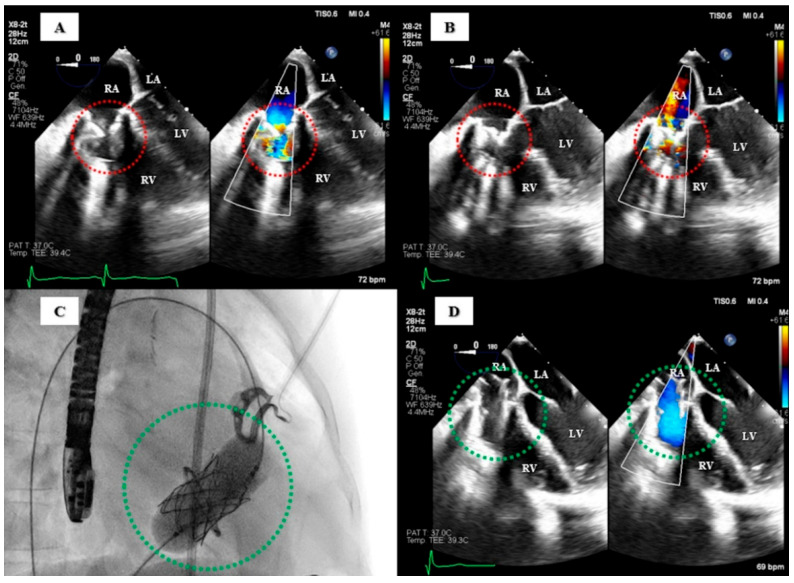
Off-label Melody TPV implantation in tricuspid position. The intra-procedural trans-esophageal echocardiography (0° mid-esophageal view) detects a degenerative biological valvular prosthesis (red circle) in tricuspid position with both a significant stenosis (A) as well as a regurgitation (B). The right lateral view angiography of Melody TPV (green circle) implantation inside the degenerated tricuspid bioprosthesis (C). After Melody TPV implantation, the intra-procedural trans-esophageal echocardiography (0° mid-esophageal view) highlights a good functioning Melody TPV (green circle) in tricuspid position without stenosis or regurgitation (D). Abbreviations. LA: Left Atrium; LV: Left Ventricle; RA: Right Atrium; RV: Right Ventricle.
